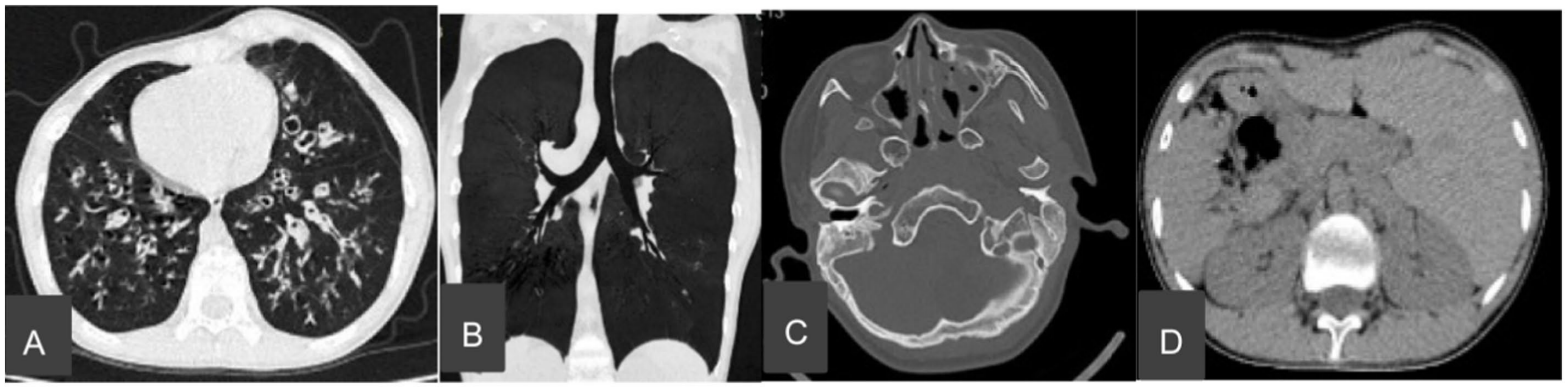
Computed tomography (CT) image of chest and nasal. (A) Bronchiectasis and bronchiolitis. (B)
